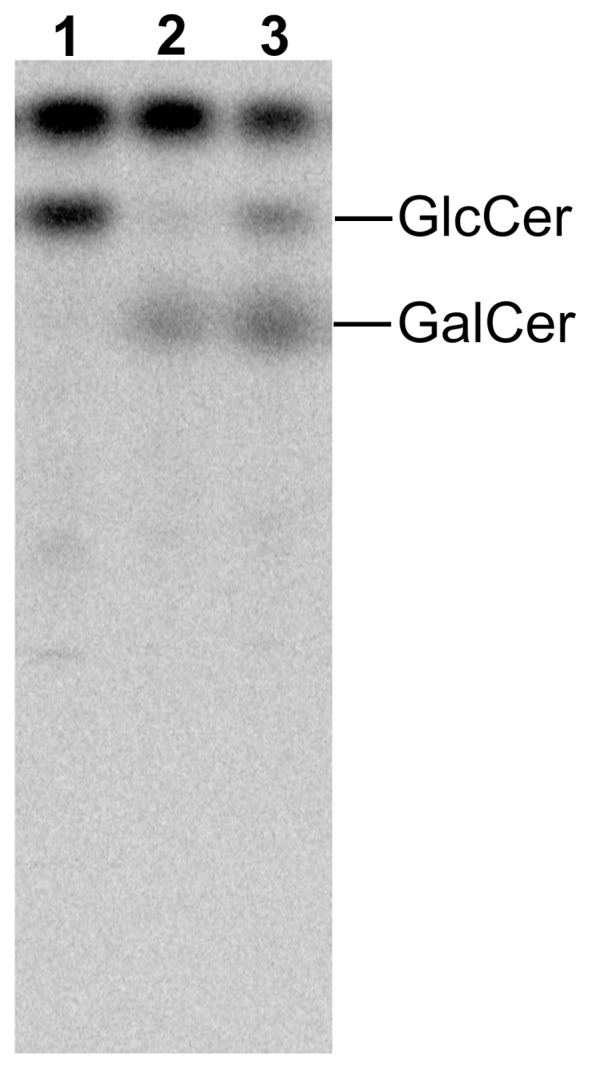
HPTLC pattern of ^14^C-serine-labelled neutral glycosphingolipids of the MDA-MB-231 cells after doxorubicin treatment. Cells were grown in medium containing 2 µC/ml ^14^C-serine for 3 h. Neutral glycosphingolipids were separated in the solvent system 2-propanol/15 M ammonia/methyl acetate/water 75/10/5/15 by vol. The plate was exposed to radiographic screen (DuPoint) for 5 days. The positions of GlcCer and GalCer standards are indicated on the right. Lane 1 – MDA-MB-231 cells grown in complete α-MEM, lane 2 - MDA-MB-231 cells grown in the presence of PPMP for 96 h, lane 3 – MDA-MB-231 cells grown at 90–100% confluence in the presence of 2.5 µM doxorubicin for 48 h.
